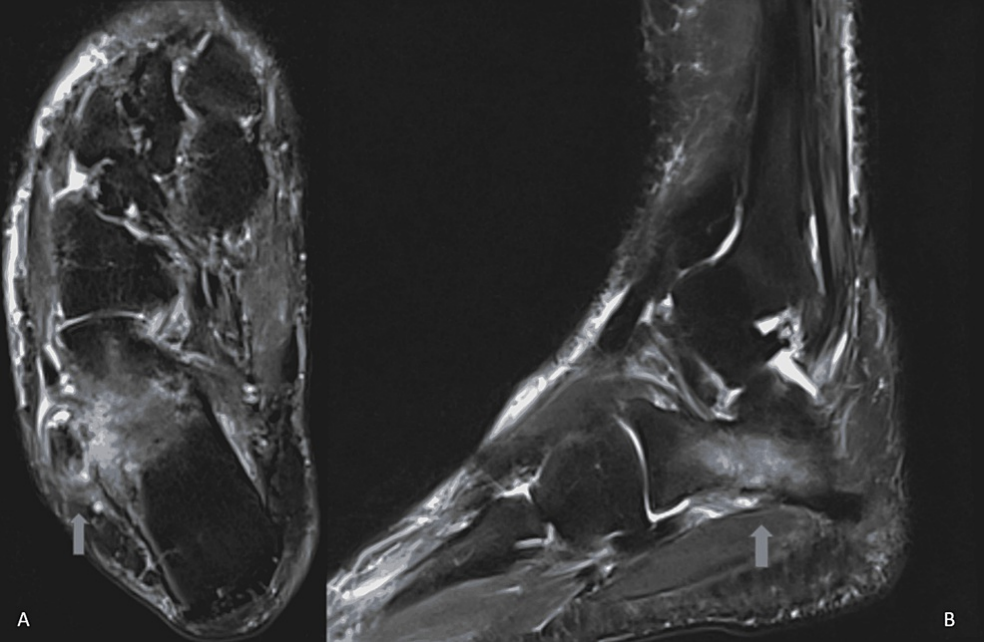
Preoperative MRI depicting an osseous lesion accompanied by diffuse bone marrow edema. A: axial plane; B: sagittal plane.
